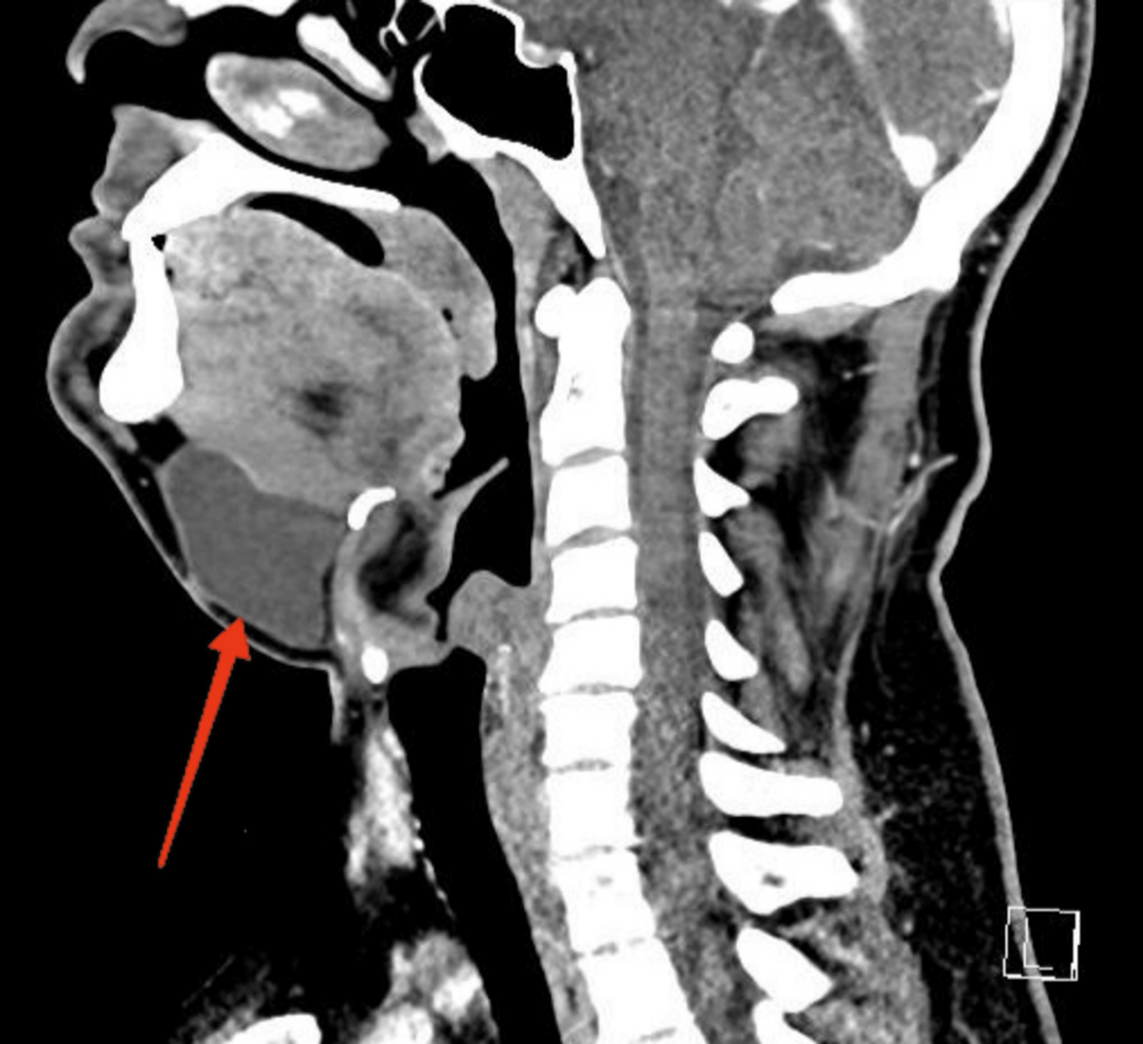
Axial CT image with intravenous contrast material showing a fluid-equivalent mass well demarcated from the surrounding parenchyma, with no peripheral enhancement. It is located medially in the submental space and cranially reaches the caudal surface of the mylohyoid muscle CT, computed tomography
